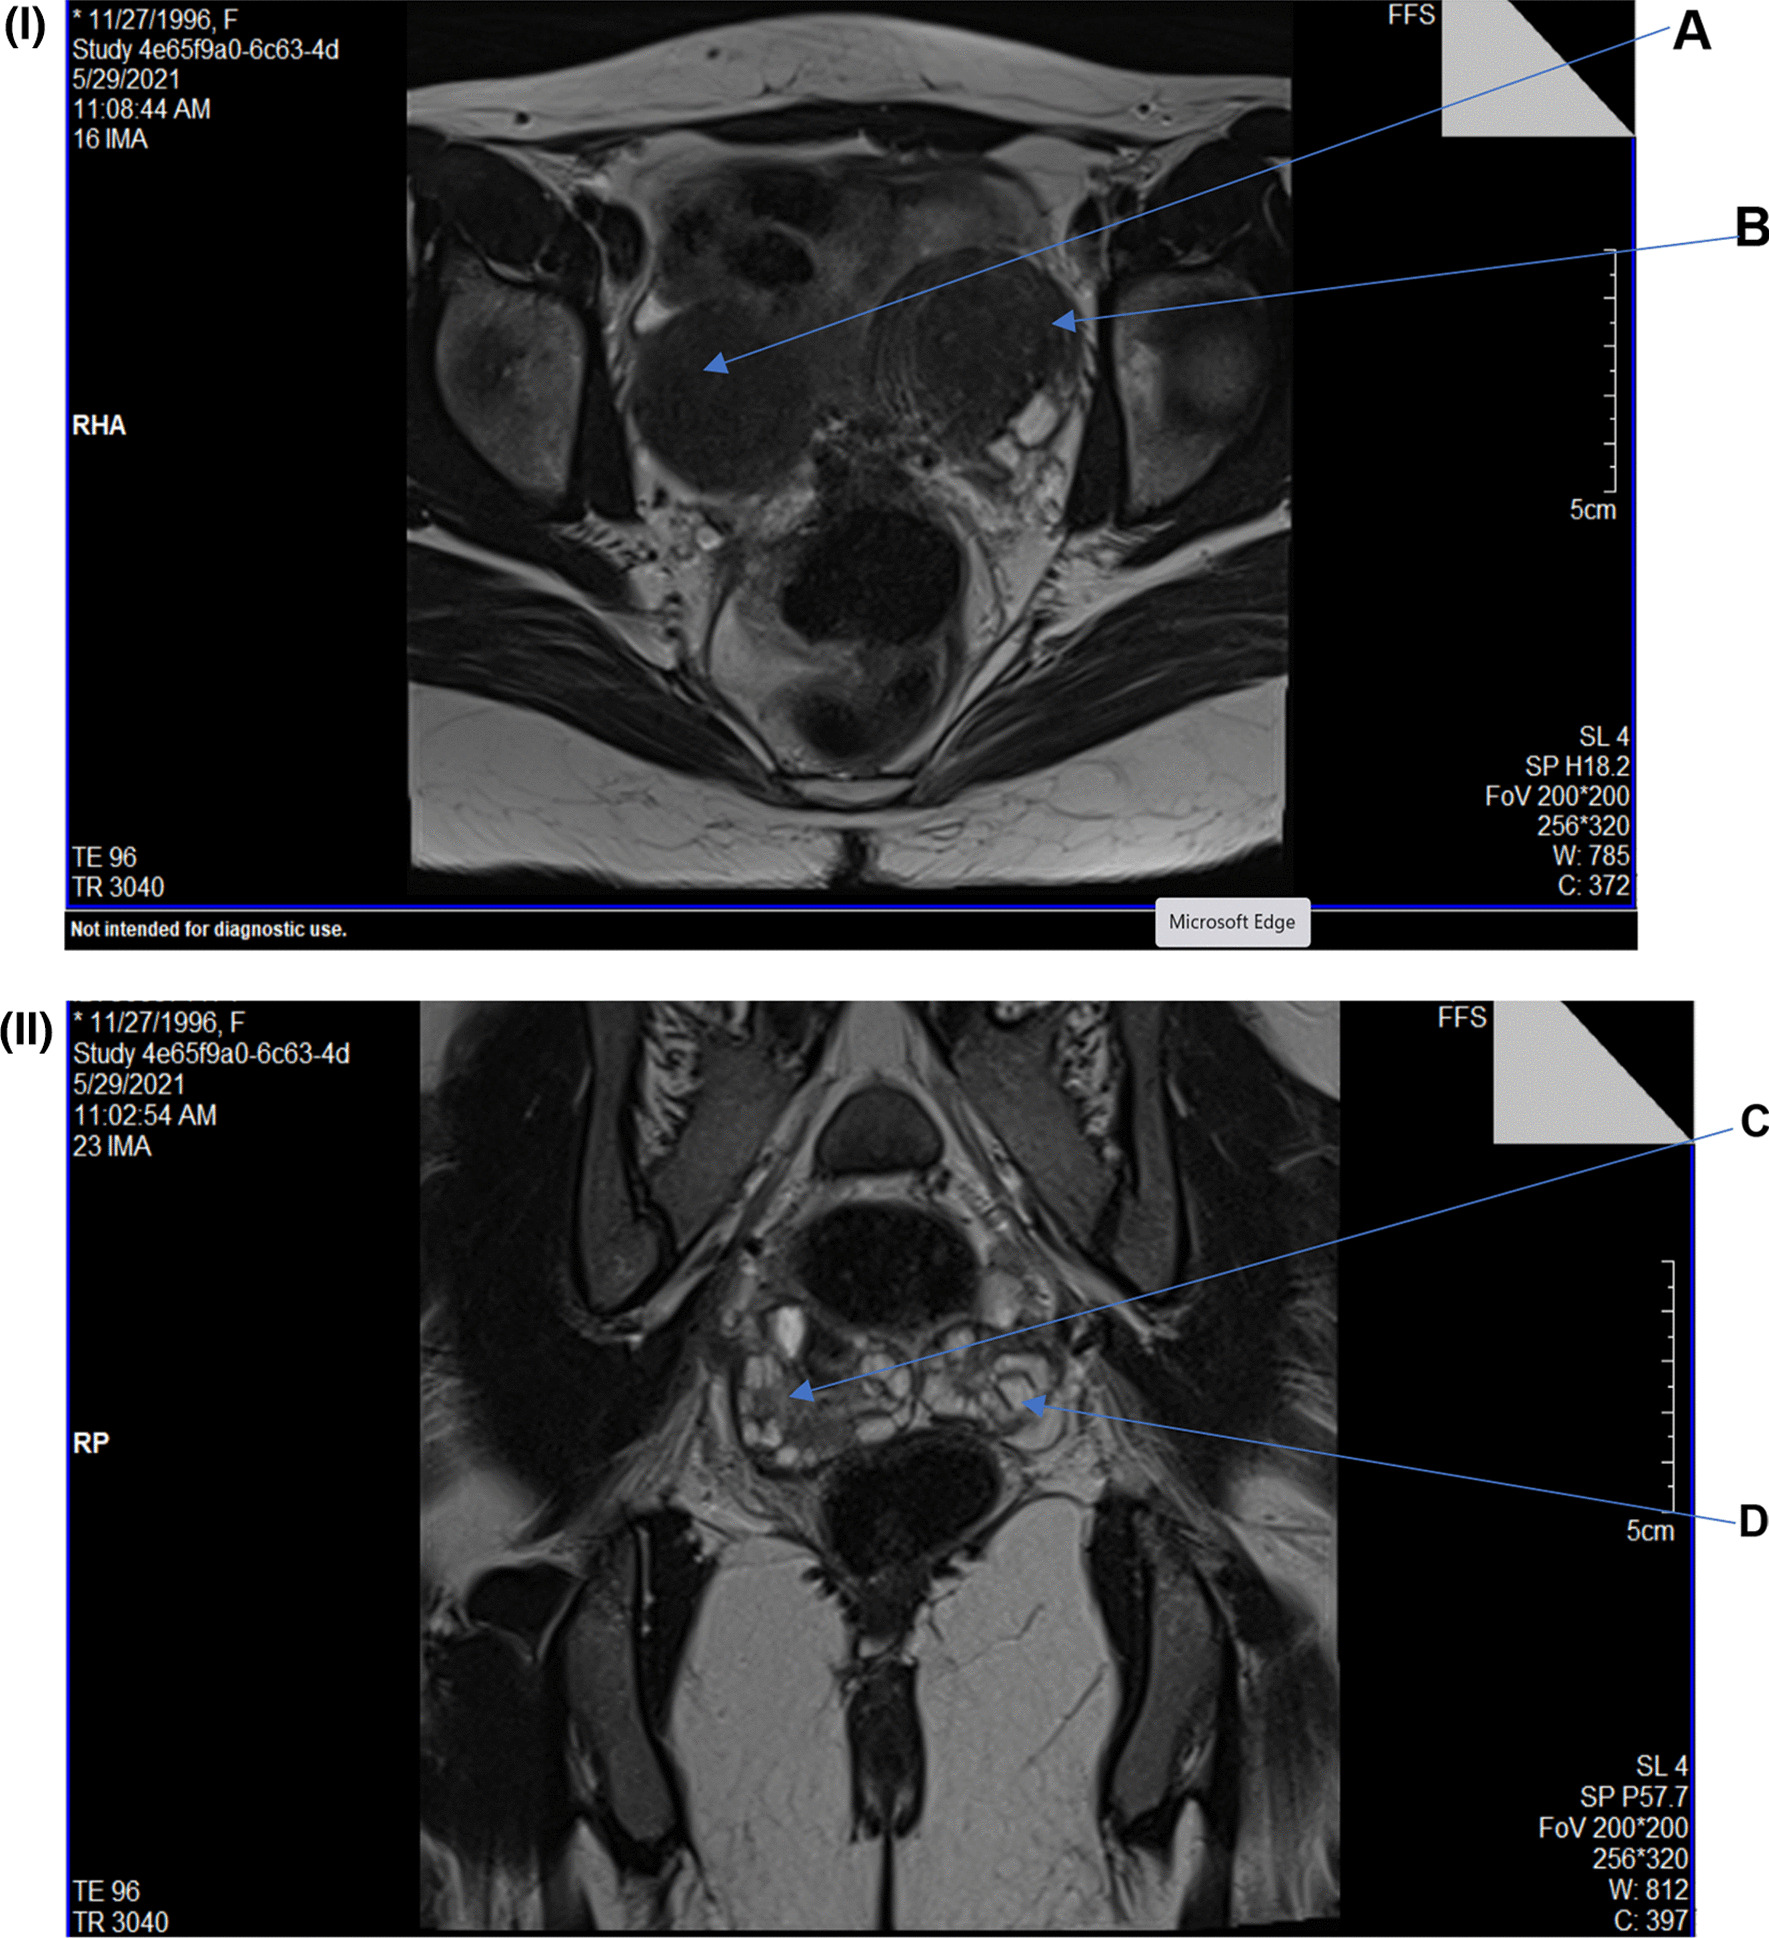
MRI of the pelvis: A Blue arrow shows uterine cavity of one cornue of bicornuate uterus. B Blue arrow shows heterogeneous myometrial signal intensity with a thickened junctional zone and indistinct endo-myometrial junction, likely adenomyosis. C, D Blue arrow shows both ovaries that were polycystic
